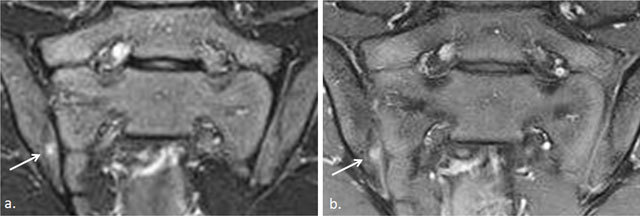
Active sacroiliitis in a 14-year-old boy with JSpA. a. Semicoronal STIR image shows a focal spot of BME at the iliac side of the right sacroiliac joint (arrow). b. Semicoronal contrast-enhanced fat-saturated T1-weighted image shows corresponding enhancement of this spot (arrow).
